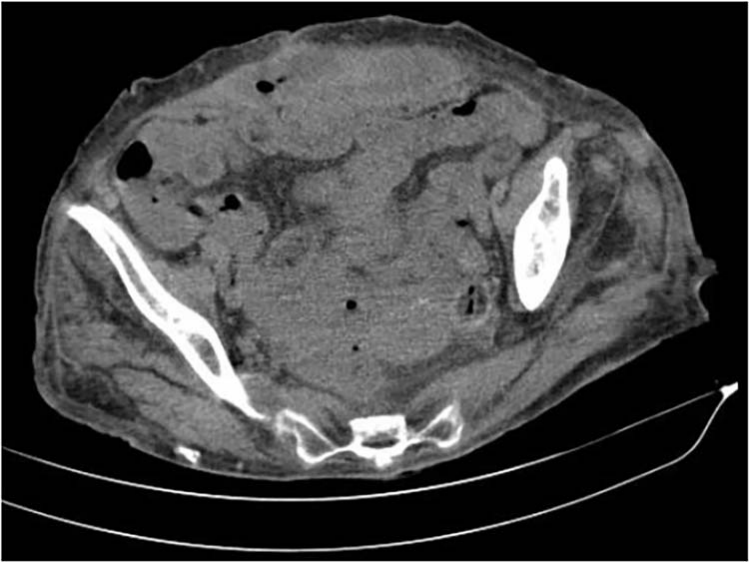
The axial CT section of the abdomen showing an abscess in the abdominal wall adjacent measured 12 × 4.0 × 13.0 cm.
